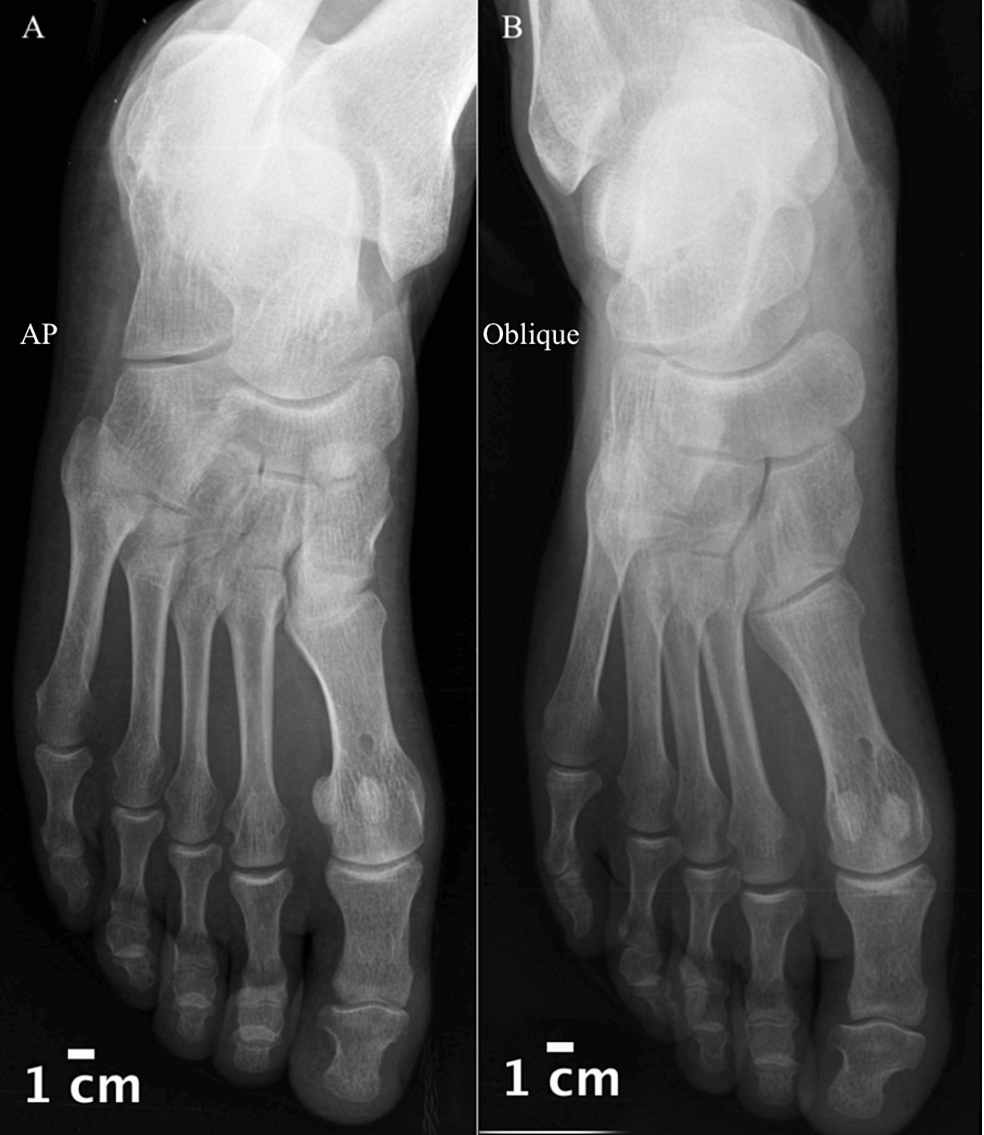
X-rays of anteroposterior (A) and oblique (B) views at one year of follow-up.
